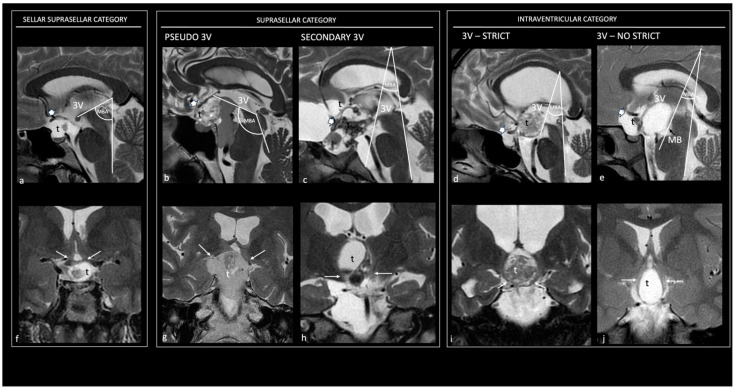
Topographical categories of craniopharyngiomas. Preoperative sagittal (a–e) and coronal (f–j) T2 magnetic resonance images show the five major topographical categories of craniopharyngiomas. Sellar–suprasellar category. Sellar–suprasellar CP (a–f): the chiasmatic cistern is occupied by the tumor, the PS is not visible, the chiasm is stretched upward (thick white arrow in (a)), the mammillary body angle (MBA) is acute (65°), and the hypothalamus (thin white arrows in (f)) is around the upper-third level of the tumor; the 3 V is free of tumor. Suprasellar category. Pseudo-third ventricle CP (b,g): the sella, chiasm cistern, and 3 V are occupied by a giant tumor; the PS is not visible, the chiasm is stretched upward (thick white arrow in (b)), the mammillary body angle is obtuse (120°), and the hypothalamus (thin white arrows in (g)) is around the upper third of the tumor. Secondary intraventricular CP (c,h): the chiasm cistern and the 3 V are occupied by the tumor, the PS is not visible, the chiasm is stretched forward (thick white arrow in (c)), the angle of the mammillary body is acute (40°), and the hypothalamus is located around the mid-third of the tumor (thin white arrows in (h)). Intraventricular category. Strictly intraventricular CPs (d,i): the chiasm cistern is tumor-free, the PS is entirely visible, the chiasm is compressed downward (thick white arrow in (d)), the angle of the mammillary body is acute (35°), and the hypothalamus is in the lower-middle third of the tumor (thin white arrows in (h)). Not-strictly intraventricular CPs (e,j): the chiasm cistern is occupied by tumor, the chiasm is compressed forward (thick white arrow in e), the angle of the mammillary body is hyperacute (30°), and the hypothalamus is located around the mid-third of the tumor (thin white arrows in (j)). 3 V, third ventricle; Ch, chiasm; MBA, mammillary body angle; t, tumor.
